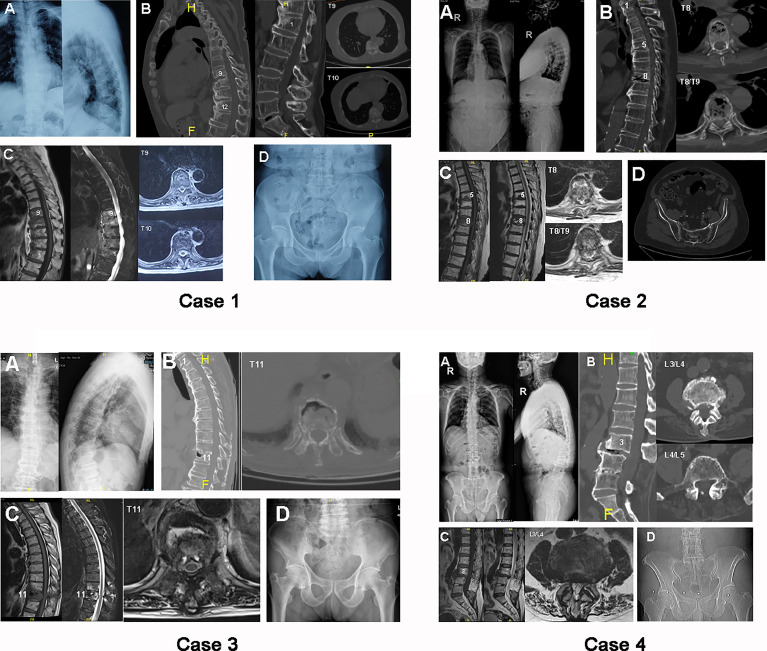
Case 1*: preoperative radiograph of case 1, a 72-year-old female. (A) Plain X-ray standing anteroposterior (AP) and lateral images. (B) Sagittal and axial computed tomography (CT) images of thoracic and lumbar segments. (C) Sagittal T1-weighted (*left*), T2-weighted (*middle*), and axial magnetic resonance (MR) scans of thoracic vertebrae. (D) Pelvic radiograph used to exclude ankylosing spondylitis (AS). *Case 2*: preoperative radiograph of case 2, an 83-year-old male. (A) Plain X-ray standing AP and lateral images. (B) Sagittal and axial CT images of thoracic segments. (C) Sagittal T1-weighted (*left*), T2-weighted (*middle*), and axial MR scans of thoracic vertebrae. (D) Pelvic radiograph. *Case 3*: preoperative radiograph of case 3, a 76-year-old male. (A) Plain X-ray standing AP and lateral images. (B) Sagittal and axial CT images of thoracic segments. (C) Sagittal T1-weighted (*left*), T2-weighted (*middle*), and axial MR scans of thoracic vertebrae. (D) Pelvic radiograph. *Case 4*: preoperative radiograph of case 4, a 73-year-old male. (A) Plain X-ray standing AP and lateral images. (B) Sagittal and axial CT images of lumbar segments. (C) Sagittal T1-weighted (*left*), T2-weighted (*middle*), and axial MR scans of lumbar vertebrae. (D) Pelvic radiograph.
